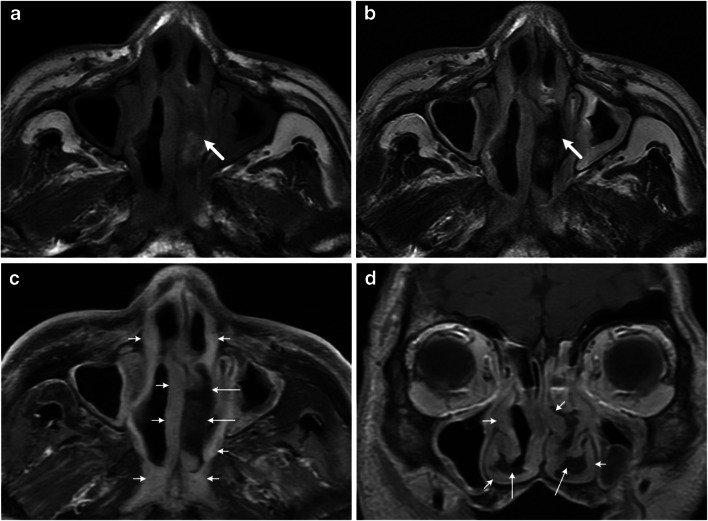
MRI features in a 67-year-old man with pathologically proven extranodal natural killer/T cell lymphoma (ENKTL) in the sinonasal region. a Axial T1-weighted MR image shows diffuse subcutaneous soft tissue thickening (small short arrows) in the bilateral nasal cavities and nasopharynx and an elongated mass (arrow) in the left nasal cavity with heterogeneous signal intensity. b Axial T2-weighted MR image shows a heterogeneous isointense tumor (arrow) with hypointense foci. c, d Axial (c) and coronal (d) contrast-enhanced T1-weighted MR images show the thickening subcutaneous soft tissue (small short arrows) with marked enhancement; no enhancement part was considered as the internal necrosis (small long arrows)
